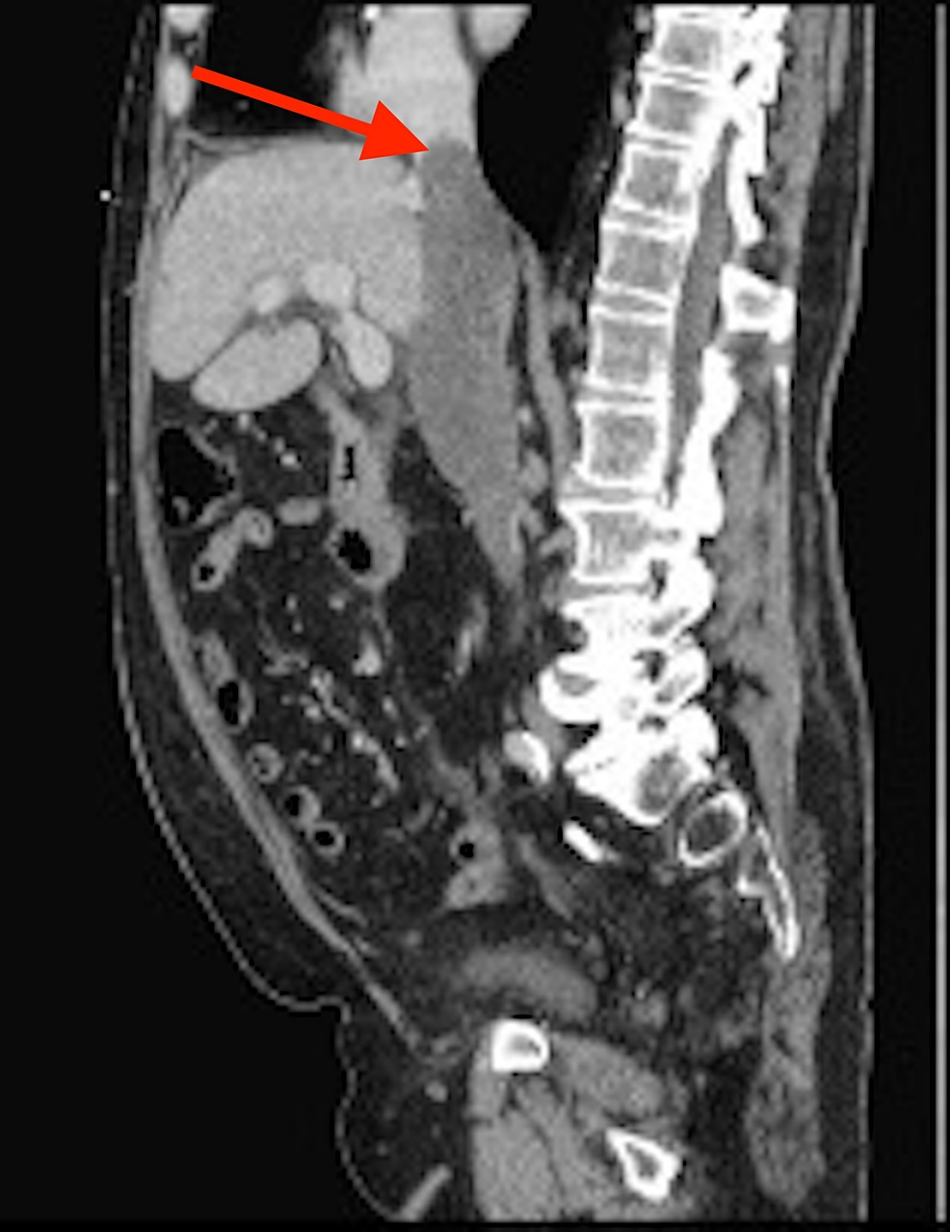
CT scan with sagittal view of inferior vena cava thrombus
